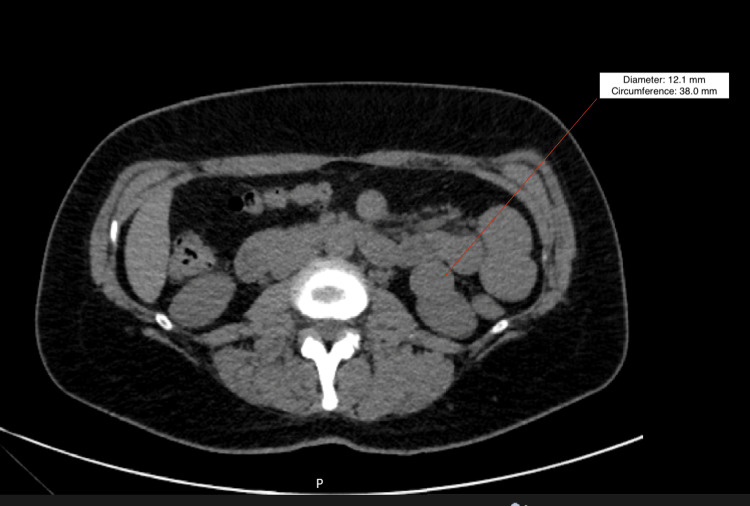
A CT scan of the abdomen and pelvis with and without contrast The red arrow indicates the lesion of the left kidney, measuring 2.5 cm x 2.2 cm.
